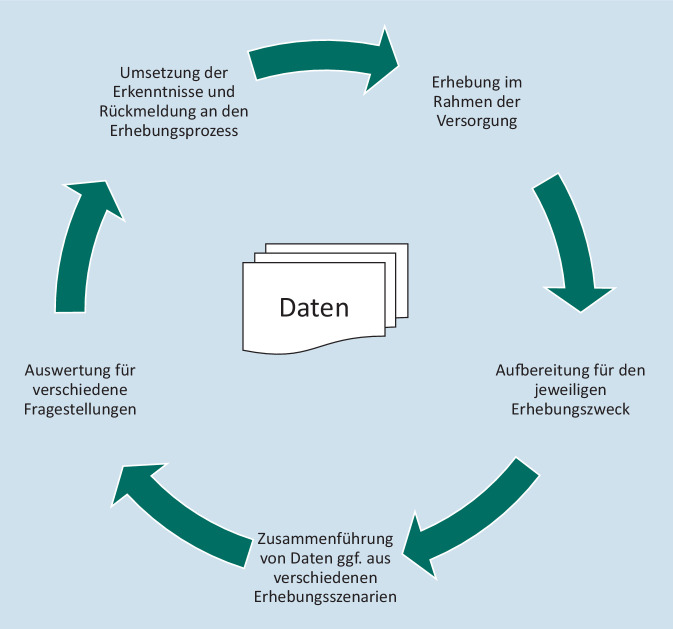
Für alle Versorgungsprozesse sollte man sich deshalb bei der Digitalisierung des Prozesses nicht nur die Fragen nach dem Datenfluss und der Datenstandardisierung für die Versorgung stellen. Vielmehr werden an diesem Punkt bereits entscheidende Weichen gestellt, die die Versorgungsforschung auf Real-World-Daten maßgeblich beeinflussen können. Im Hinblick auf die Entwicklung des Europäischen Gesundheitsdatenraums sollte bei den nationalen Überlegungen immer auch zusätzlich ein Abgleich mit europäischen Entwicklungen erfolgen. Schaut man sich das Beispiel der Labordaten an, so wird die Versorgungsforschung auf europäischer Ebene aktuell das Problem überwinden müssen, dass in Europa verschiedene Kodiersysteme verwendet werden. Möchte man Daten aus einem Land, das LOINC für die Kodierung verwendet, mit Daten aus einem anderen Land, das NPU für die Kodierung verwendet, zusammenführen, so muss ein Mapping erfolgen. Dies kann in großen Datenmodellen, wie z. B. OMOP^18^, hinterlegt werden. Geht aber mit dem Mapping ein Informationsverlust einher, so wird dieser die gesamte Datenauswertung betreffen. Für die Analyse wird das Kodiersystem mit der niedrigsten Detailtiefe als „kleinster gemeinsamer Nenner“ gewählt werden müssen. Möchte man Daten aus verschiedenen Ländern zusammenführen, die alle in wenig detaillierten Kodiersystemen erhoben wurden, so wird selbst ein Mapping der verschiedenen Kodiersysteme auf ein feiner granuläres Kodiersystem wie SNOMED CT keine detaillierten Analysen möglich machen. Durch den möglicherweise zusätzlichen und unterschiedlichen Informationsverlust des jeweiligen Mappings wird dieser im Zweifel sogar noch größer, als wenn ein direktes Mapping der beiden Kodiersysteme der Länder erfolgt. Möchte man aber in Europa Daten zusammenführen, die mit einer Vielzahl an unterschiedlichen Kodiersystemen erhoben wurden, wie aus dem Beispiel der Operationen in Abb. 1 ersichtlich, so kann das Mapping auf ein einheitliches Kodiersystem ggf. als einzige praktikable Lösung in Sekundärdatenauswertungen herangezogen werden.
